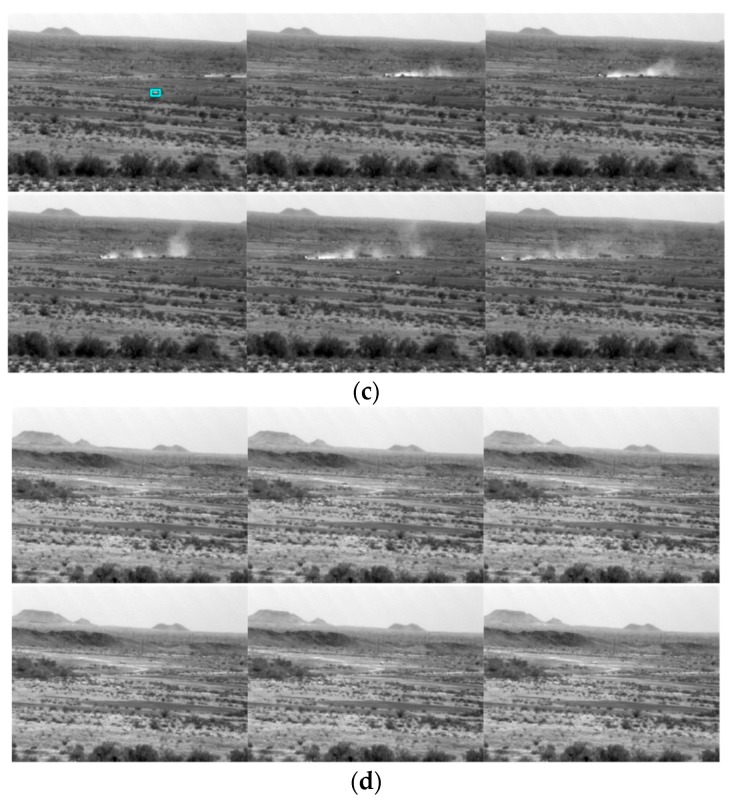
Tracking results for frames 1, 63, 126, 189, 252, and 315 in the PCE full (optical videos) case. The vehicle is SUV. Coded aperture compresses every five frames into one. (a) 1000 m; (b) 2000 m; (c) 2500 m; and (d) 3500 m.
